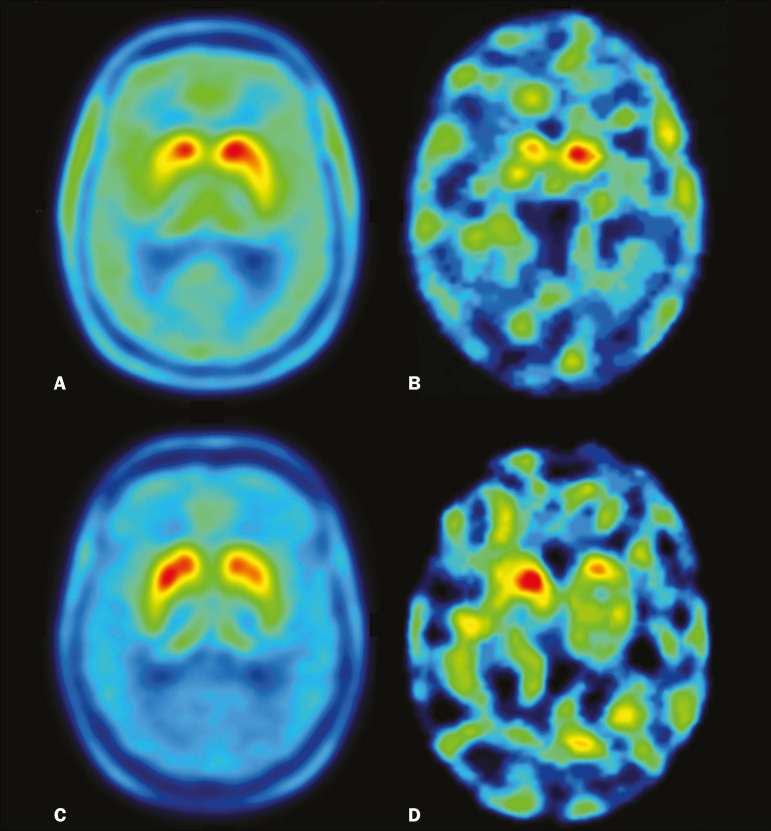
Representative transverse ^18^F-FDOPA PET image (left) and concordant ^99m^Tc-TRODAT-1 SPECT image (right) of patients with PD (A,B: subject 7; C,D: subject 6). A,B: Note the markedly low tracer uptake in the right putamen, the uptake also being low, to a lesser degree, in the left putamen. C,D: Inversely, tracer uptake was markedly low in the left putamen and low, to a lesser degree, in the right putamen.
